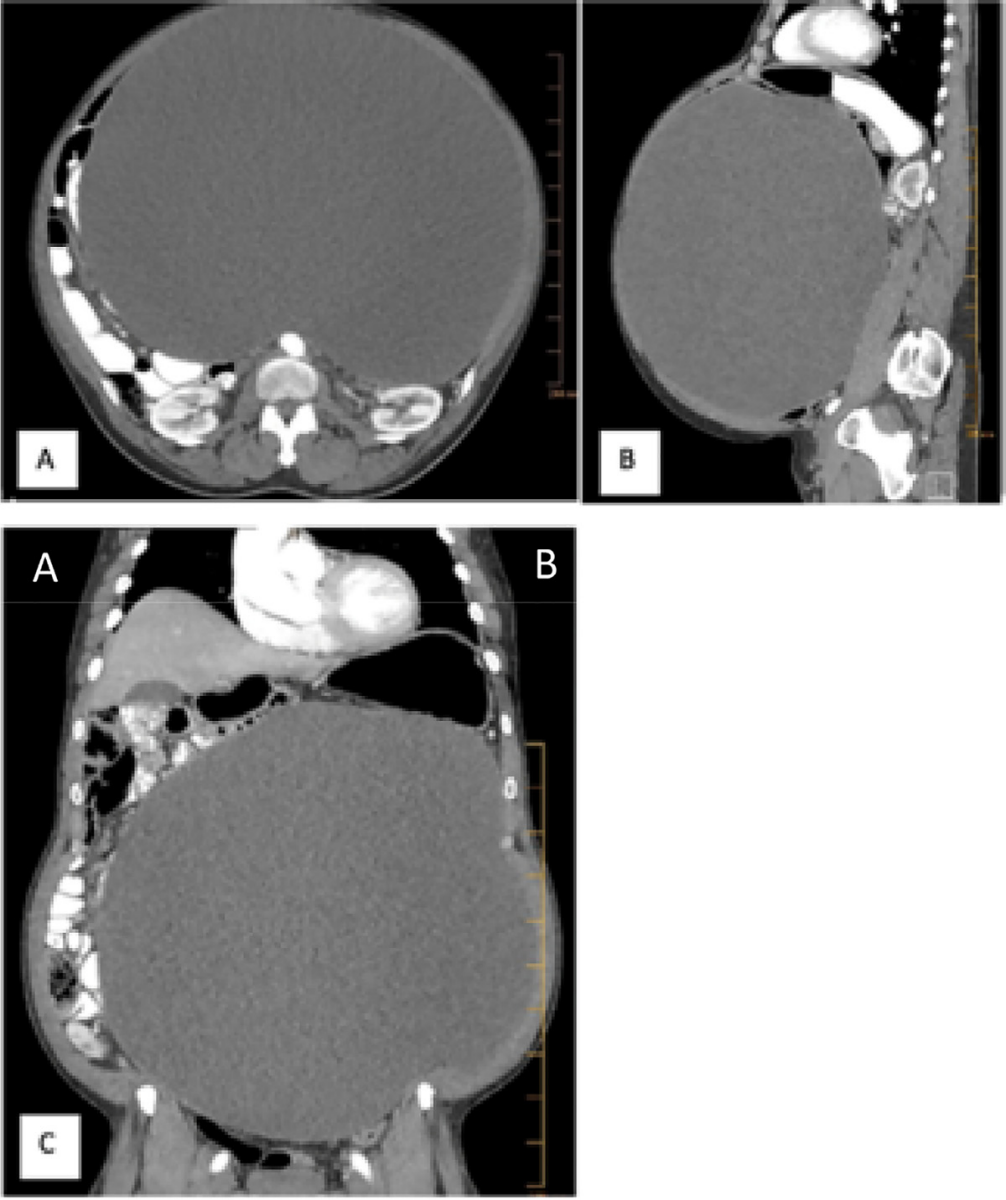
Contrast-enhanced axial (A), sagittal (B), and coronal (C) CT images showing a huge low attenuation abdominopelvic with attenuation value of 16-25 HU. No solid enhancing components or calcifications within the mass. The lesion displaces the surrounding structures and causes contour bulge of the abdominal wall.
